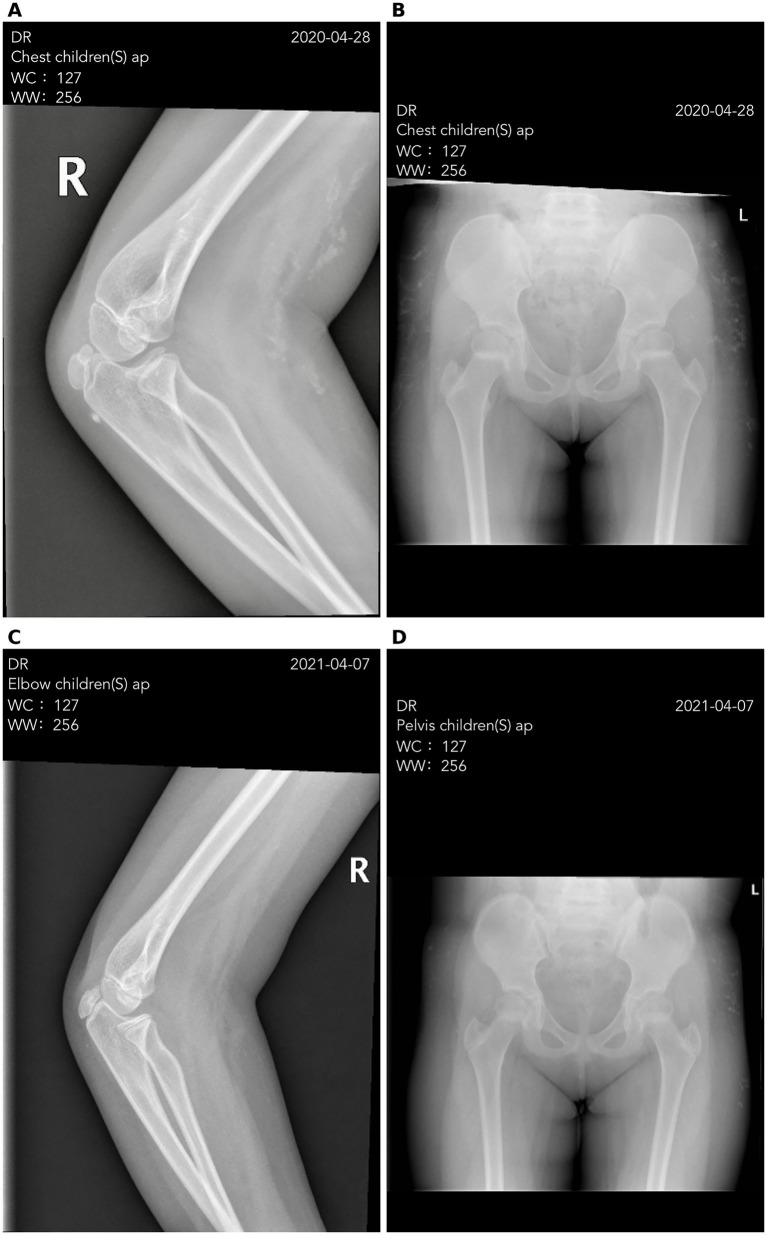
Radiographic regression of calcinosis following TNF-α blockade in an NXP2-positive patient. (A,B) Baseline radiographs (April 28, 2020) show periarticular soft-tissue calcific deposits (arrows). (C,D) Follow-up radiographs after prolonged TNF-α inhibition (April 7, 2021) demonstrate reduced maximal diameter and radiographic density of corresponding deposits (arrows). Radiographic improvement was defined as ≥20% reduction in the maximal diameter of the largest calcific deposit and was independently assessed by two radiologists blinded to clinical outcomes.
